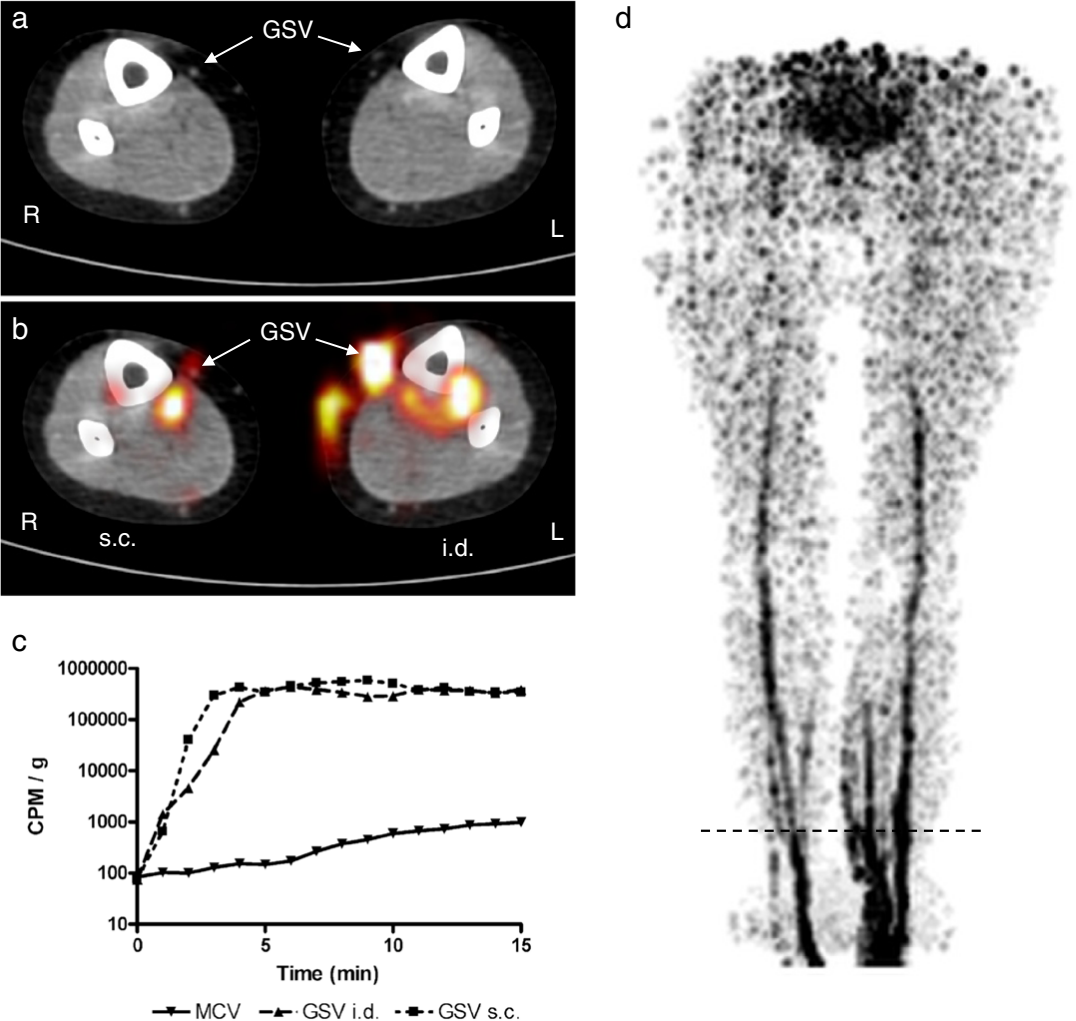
Results for subject 1. A 58-year-old woman. ^18^F-FDG (5 MBq in 0.1-mL isotonic saline) was injected into the dorsal skin of each foot subcutaneously (s.c.) on the right and intradermally (i.d.) on the left. (a) Axial low-dose CT of the distal lower leg. The great saphenous veins (GSV) are indicated by arrows. Scan level corresponds to the horizontal dashed line in (d). (b) Axial PET superimposed on CT showing ^18^F-FDG activity in a deep vein in the right lower leg. In the left lower leg, ^18^F-FDG activity is visible both superficially in the GSV and deeply in a posterior tibial vein with a communicant vein. (c) Time-activity curve for blood samples from the medical cubital vein (MCV) and GSVs with either i.d. or s.c. depot placement. Note the logarithmic scale on the *y*-axis due to large differences in measured activity. (d) Thick-slab maximum-intensity-projection PET image of the lower body showing the activity distribution of ^18^F-FDG.
